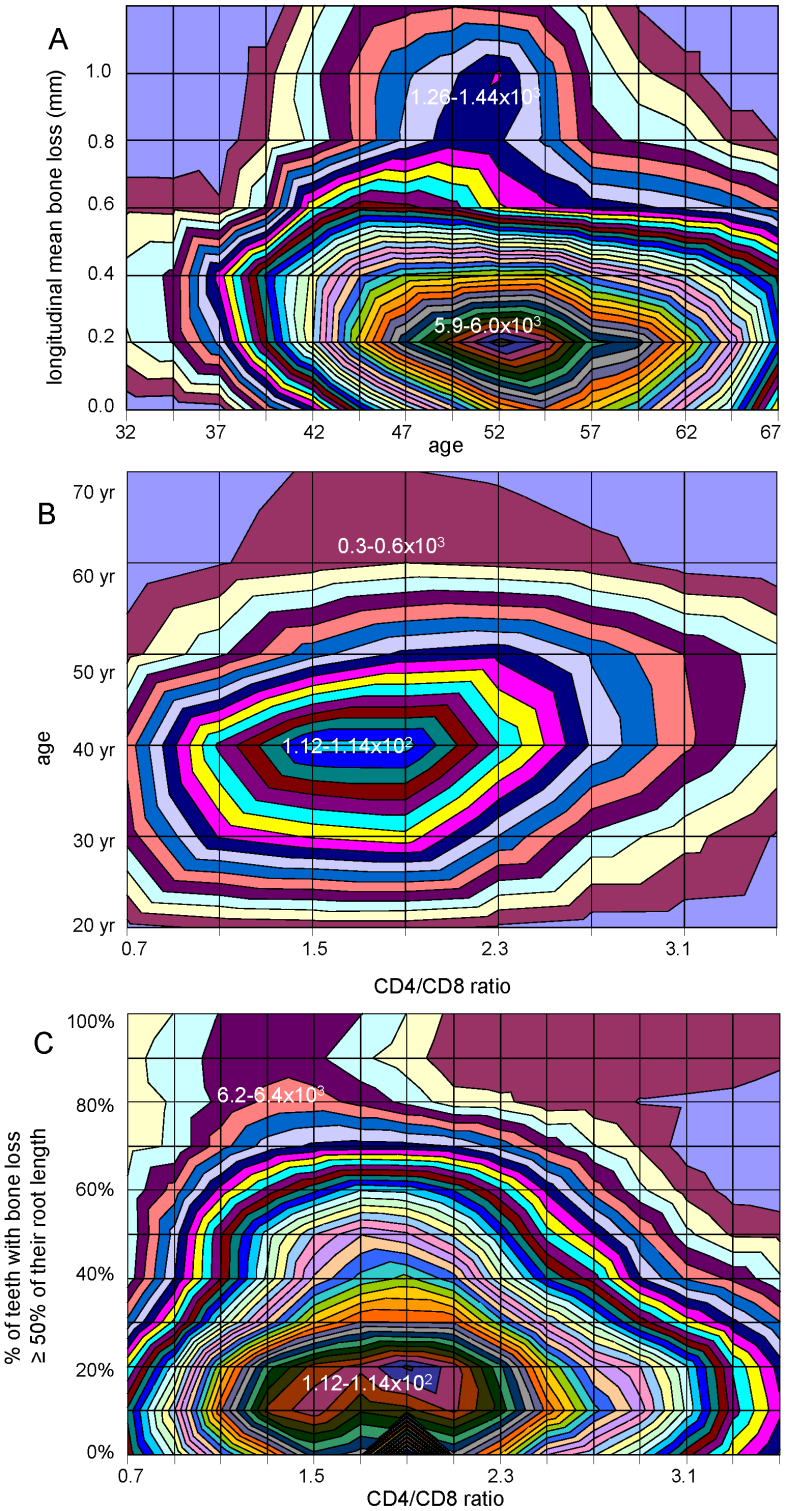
Bivariate kernel density estimation (KDE) for some selected parameters. (A) Contour plot for bivariate KDE of longitudinal radiographic bone loss level (sample-1) in relation to age: this topographical-like plot shows a main cluster with 0.2 mm longitudinal bone loss and a small cluster with almost five times greater bone loss. (B) Contour plot for bivariate KDE: By estimating probability density for CD4/CD8 ratio by age (sample-2), we see two clusters although not separated distinctly, at modes of 1.5 and 1.9. (C) Contour plot for bivariate KDE: By estimating probability density for CD4/CD8 ratio (sample-2) by disease severity (% of teeth with bone loss ≥ of 50% of their root length), we reveal two distinct clusters of patients, with modes at *x* values of 1.5 and 1.9.
